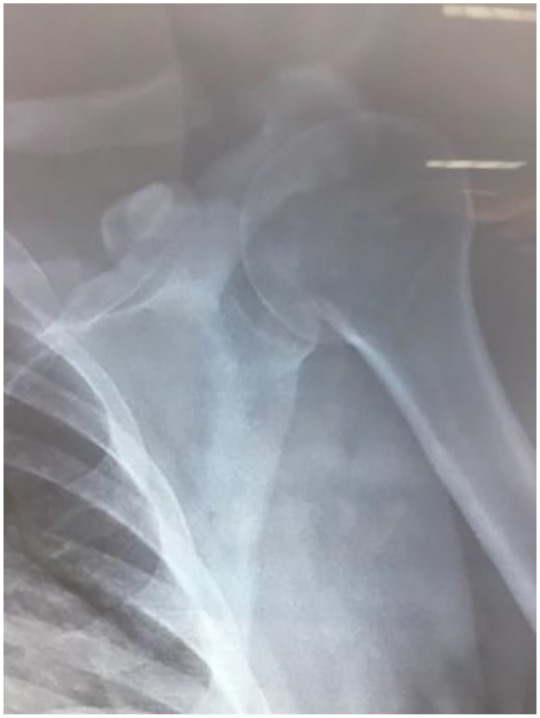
Post-reduction X-ray with restoration of the normal position of the humeral
